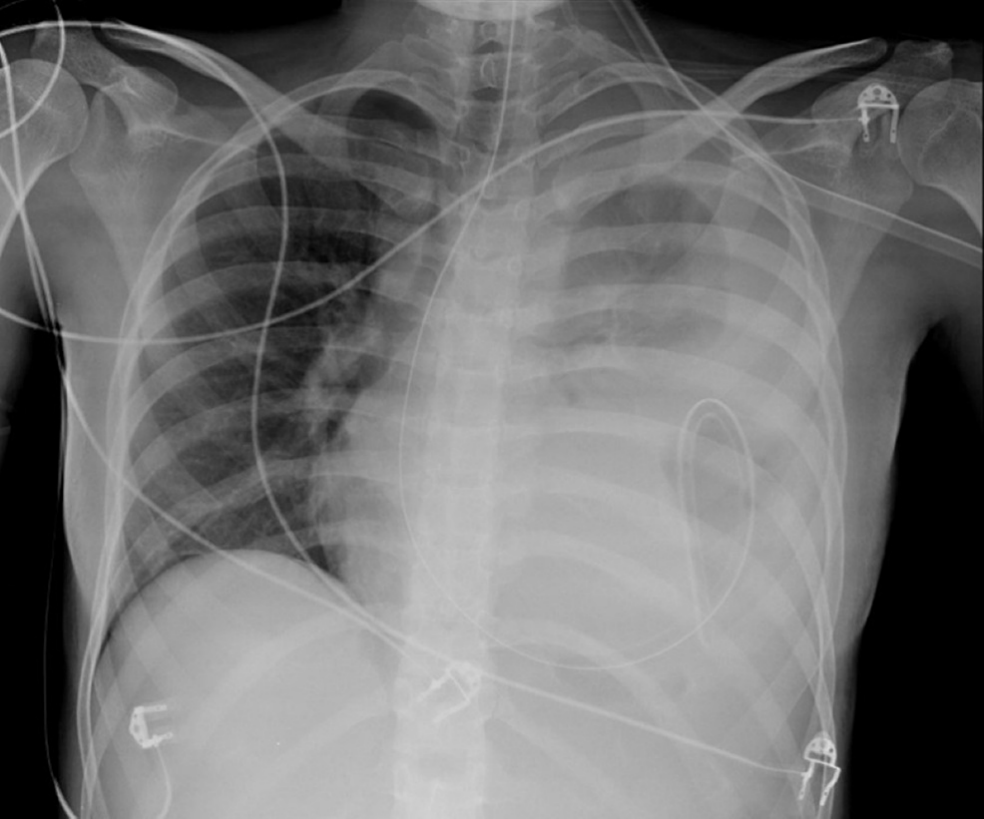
Second chest X-ray Preoperative chest X-ray showed air-fluid levels within the left hemithorax and the nasogastric tube above the diaphragm.
